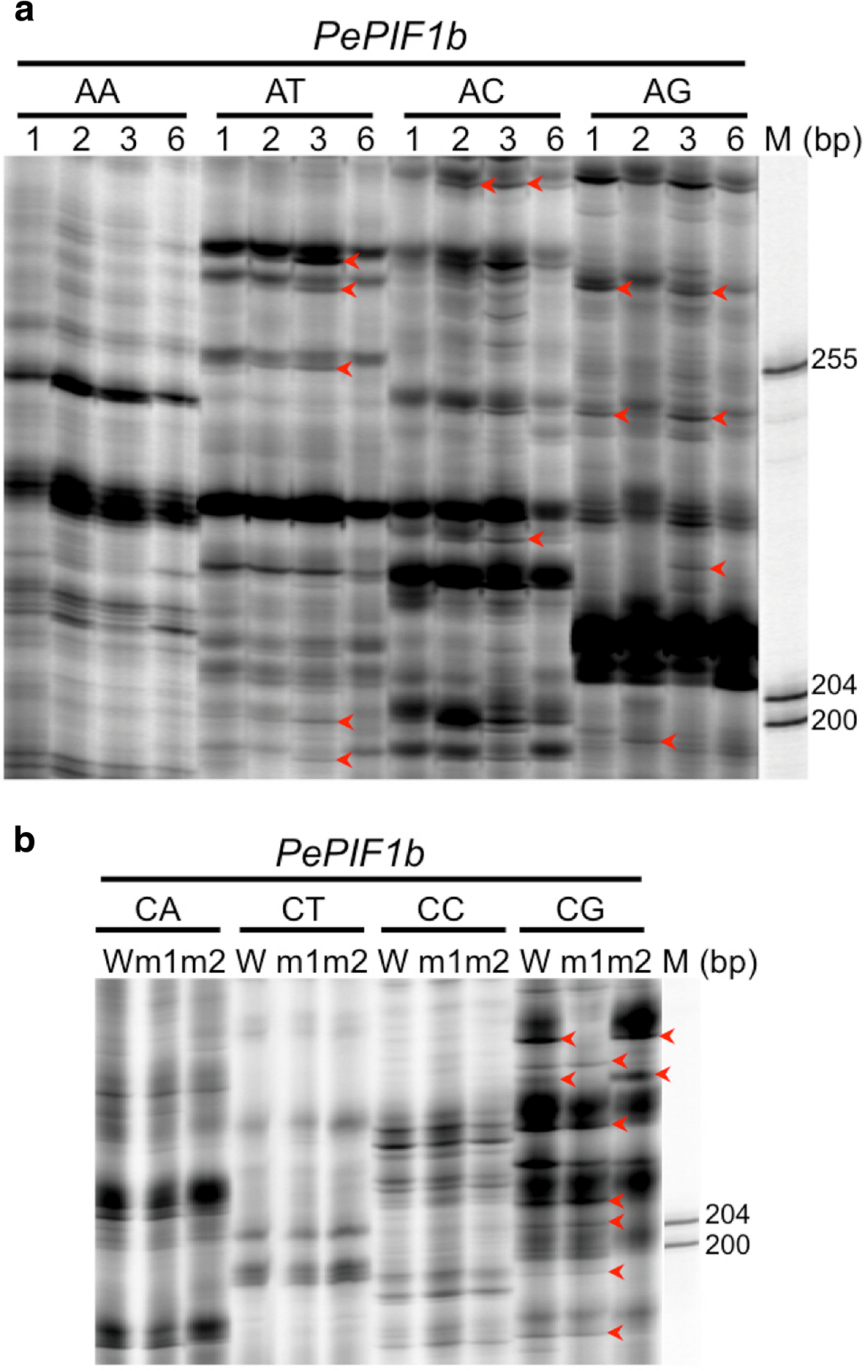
Transposon display profile of *PePIF1* in various PLB generations of KHM1219 (a) and the wild-type and somaclonal variants of KHM2180 with various pigmentation patterning (b). a Seedlings from various PLB generations of KHM1219 (N1, N2, N3 and N6) are labeled 1, 2, 3, and 6. b Wild type and somaclonal variants of KHM2180 are labeled W, m1, and m2. The DNA size markers are indicated as “M” on the right and left side of the gel. AA, AT, AC, AG, CA, CT, CC, and CG indicate the second selective primers used for PCR amplification. The red arrowheads indicate the differentially amplified fragments with a new band
